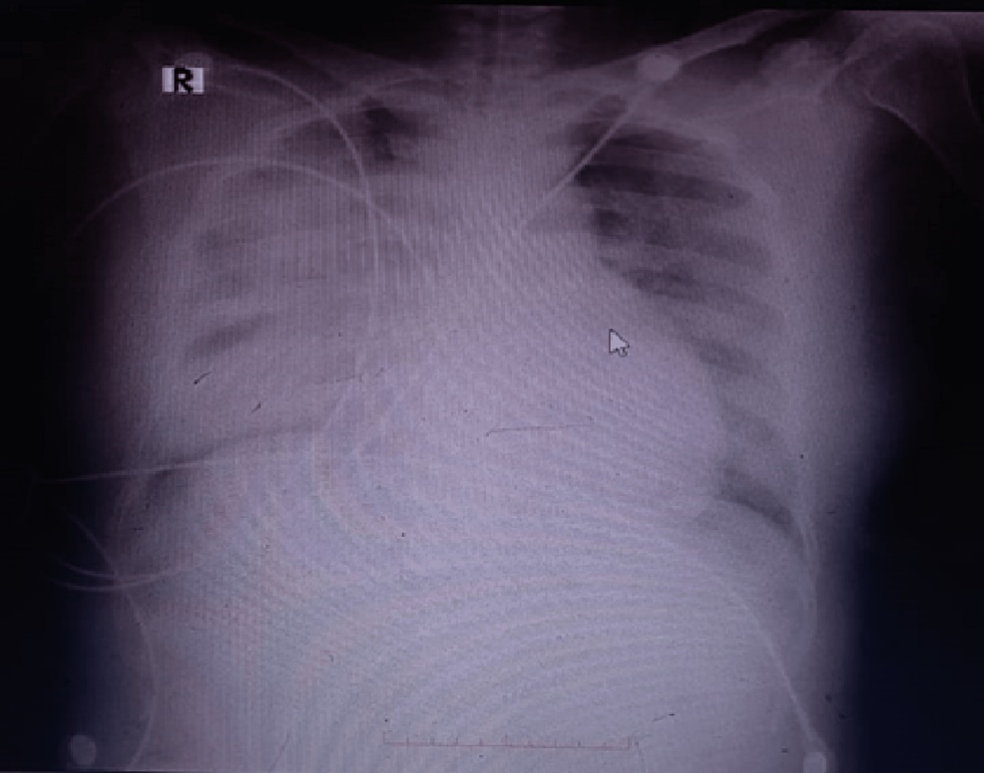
Chest x-ray taken on October 17, 2022
